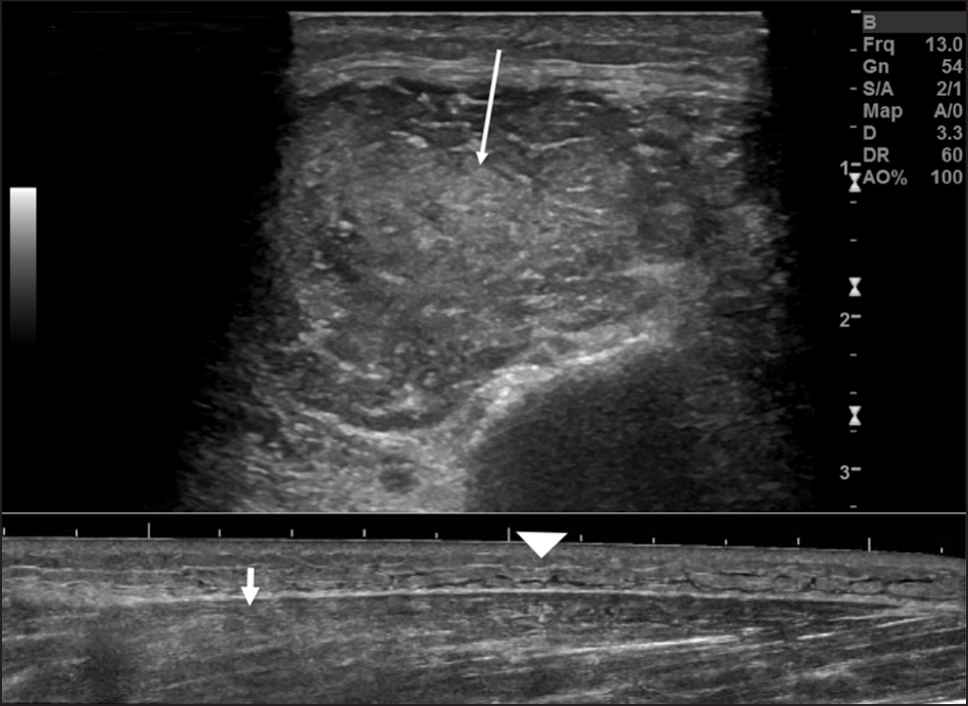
Ultrasound exam. *Top:* Transverse image of the peroneal muscle compartment shows increased echogenicity (long arrow), compatible with myositis. *Bottom:* Longitudinal image with muscle swelling (short arrow) and subcutaneous edema (arrowhead) in the proximal one-third of the lower leg.
